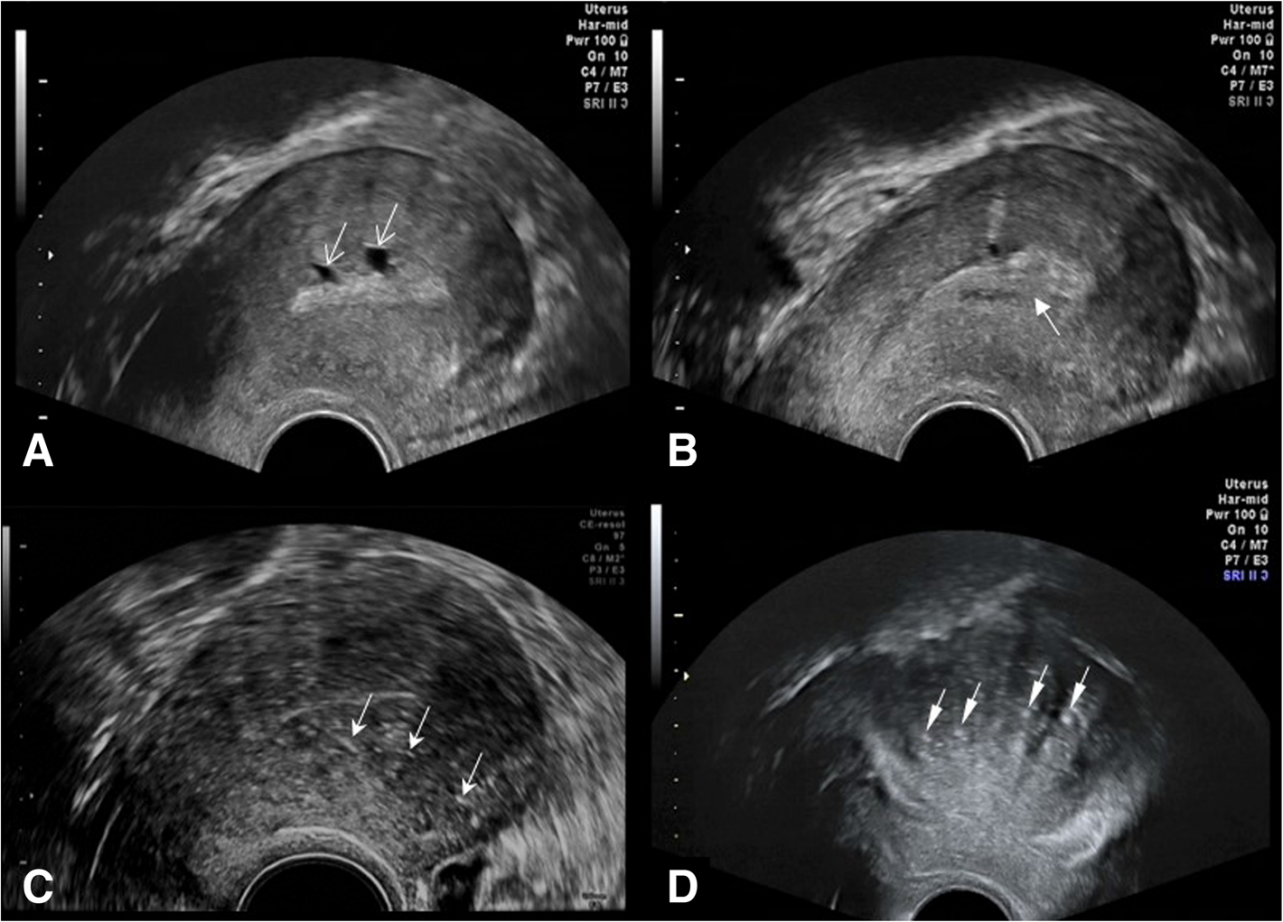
Direct and indirect imaging features of adenomyosis on ultrasound. a Small posterior wall myometrial cysts (open arrows). b Poorly defined endo-myometrial interface (solid arrow). c Diffuse myometrial heterogeneity with hyperechoic linear striations (three arrows). d Diffuse asymmetric widening of the posterior myometrial wall with hyperechoic nodules (four arrows)
